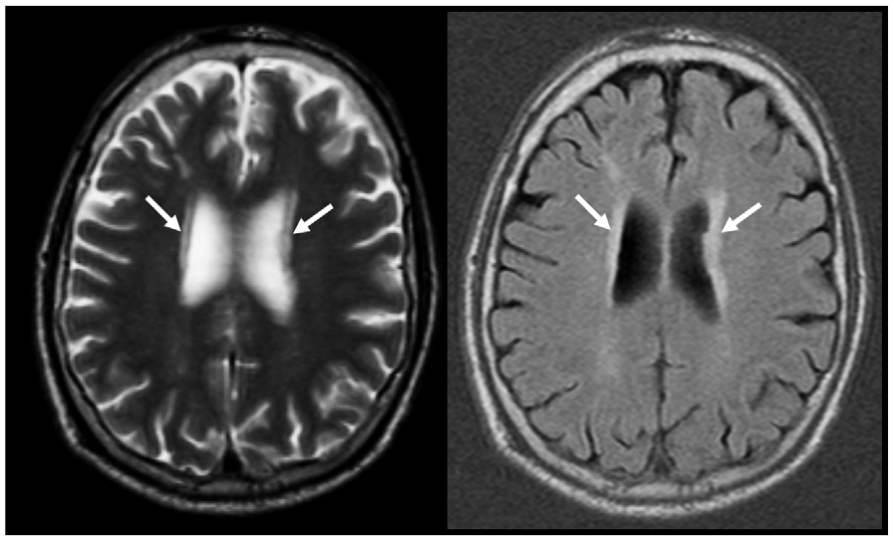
Transverse T2-weighted TSE and fluid-attenuated inversion recovery images showing WMLs, mainly located adjacent to the ventricles (arrows).
